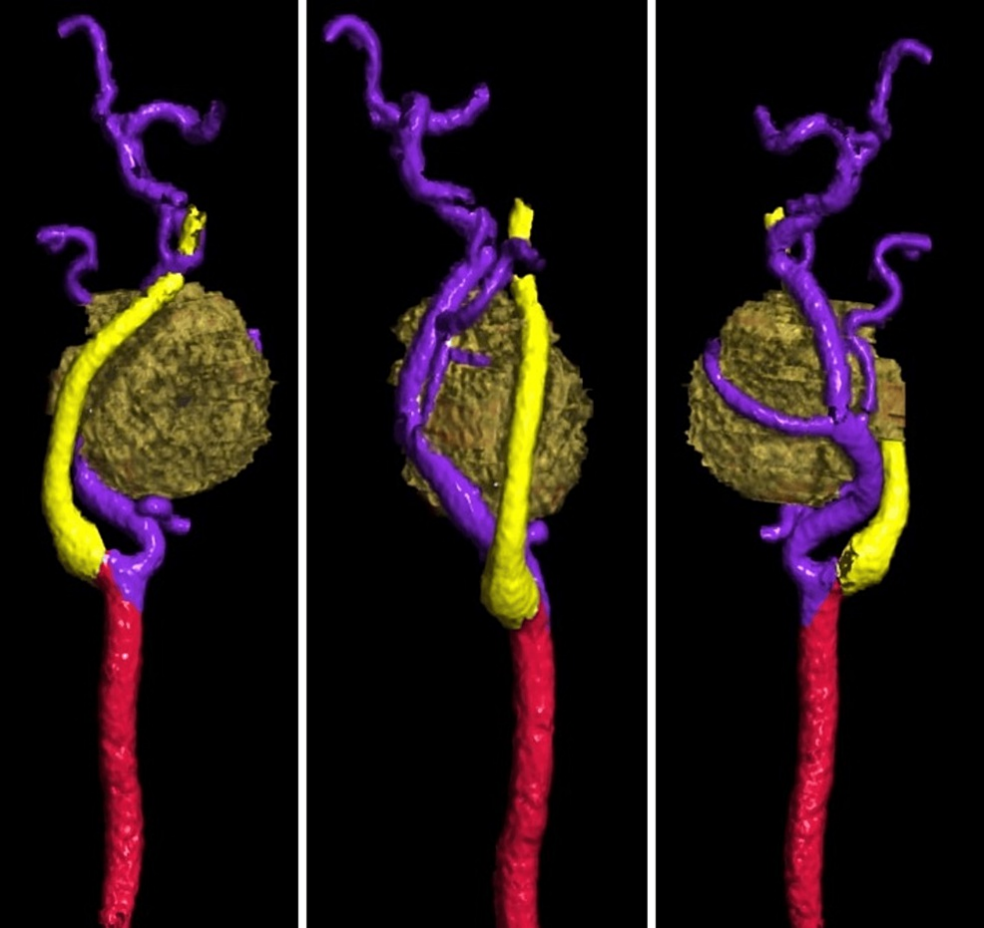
3D color-coded image of left carotids from CTA of the neck (Siemens Healthineers) A soft tissue density lesion was observed in the left carotid space with its relation to the left internal carotid artery (yellow color-coded), left external carotid artery (purple color-coded), and left common carotid artery (red color-coded). CTA: Computed tomography angiography.
